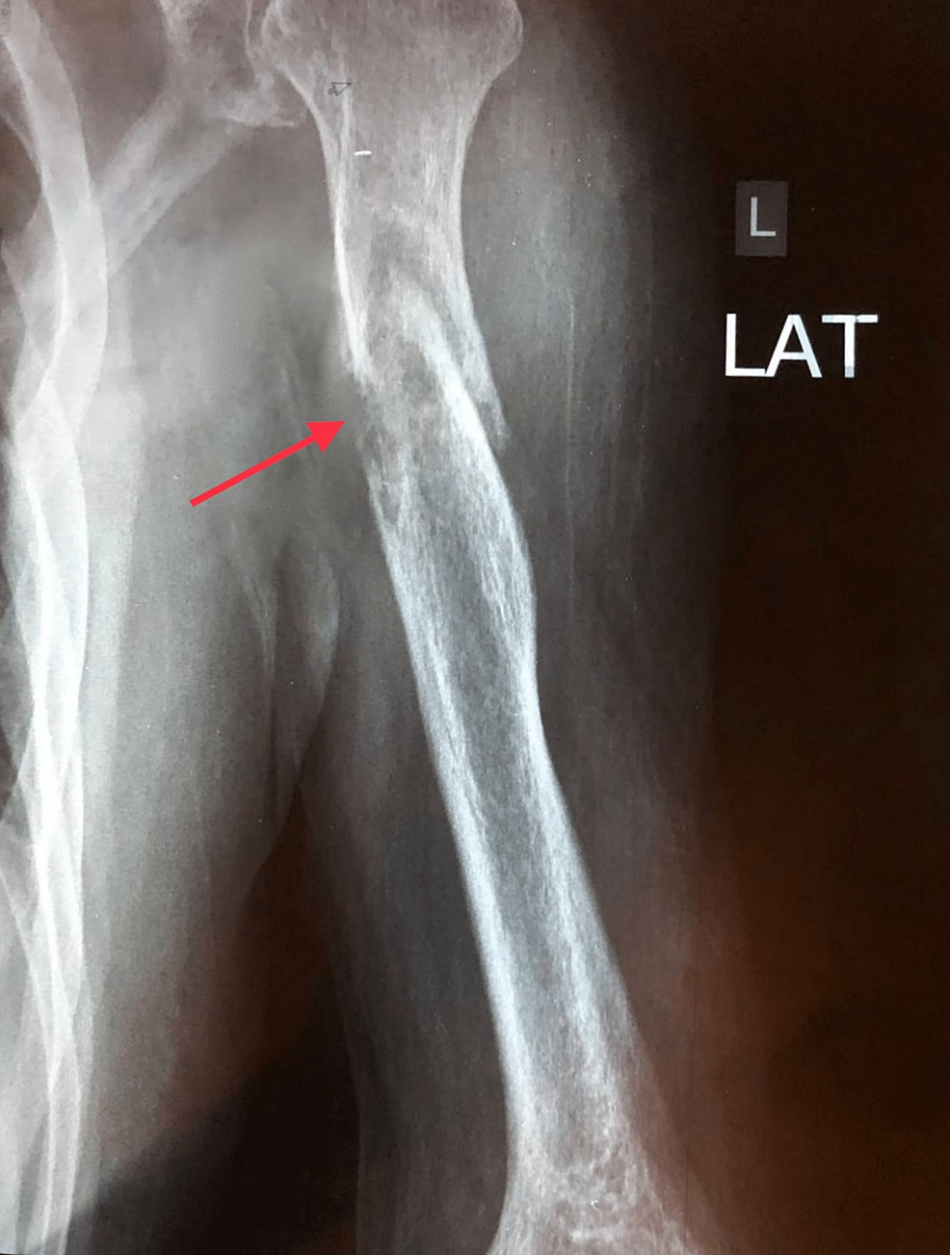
Plain radiograph showing pathological fracture in the left humeral shaft.
